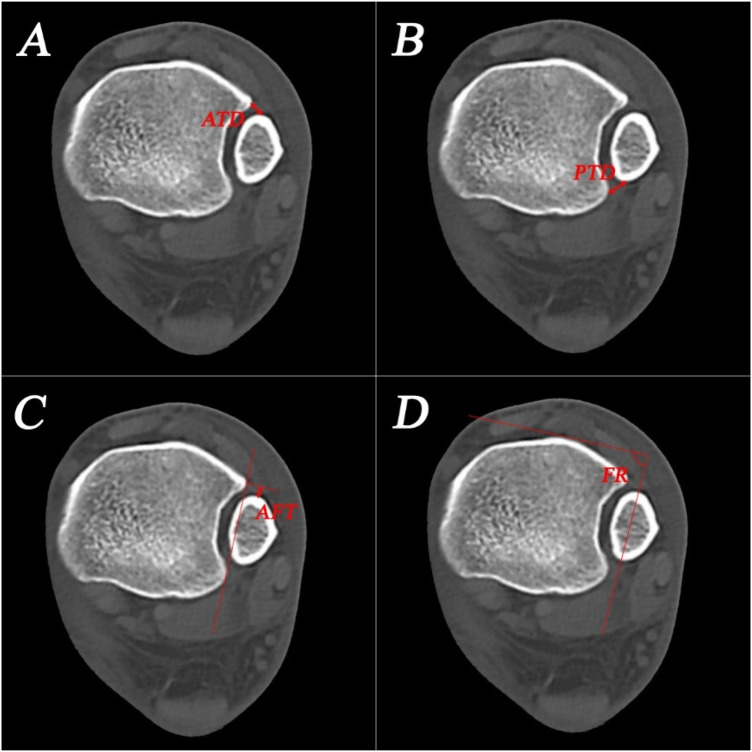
Measurements for evaluating syndesmotic reduction on CT images. (A) anterior tibiofibular distance (ATD); (B) posterior tibiofibular distance (PTD); (C) anteroposterior fibular translation (AFT); (D) fibular rotation (FR).
